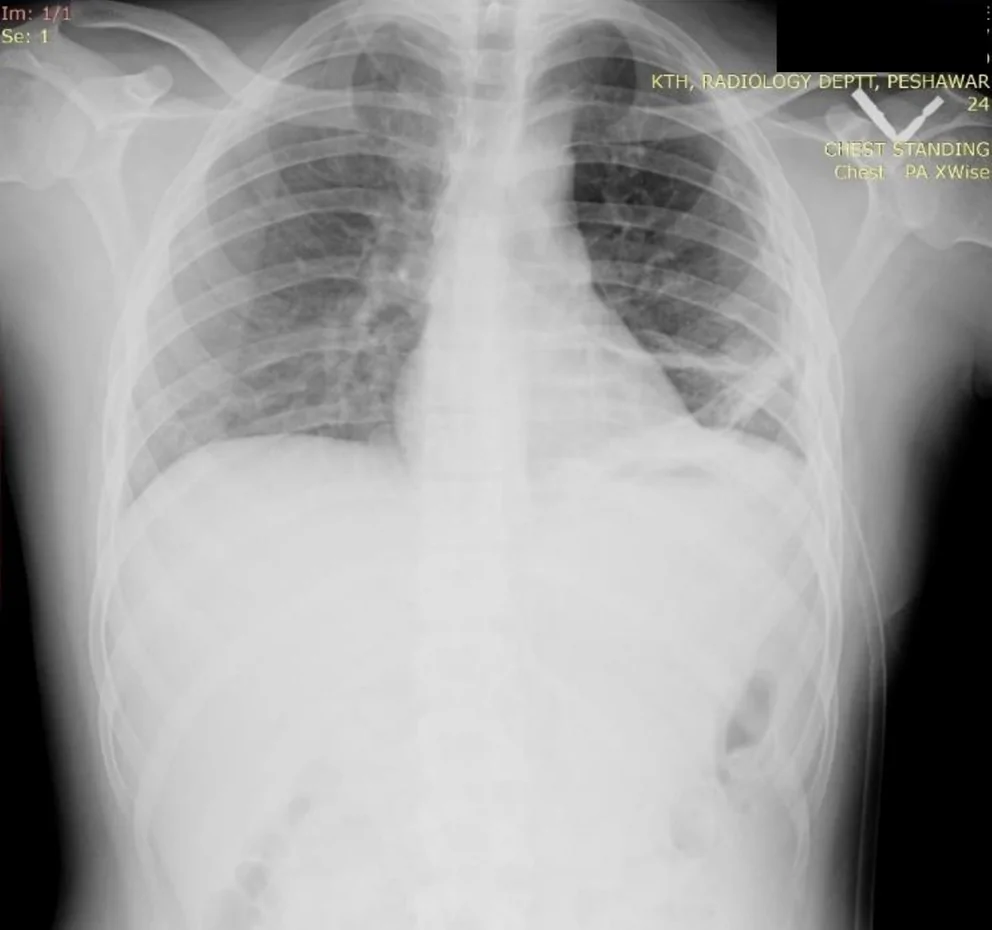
Chest radiograph following tube thoracostomy.
